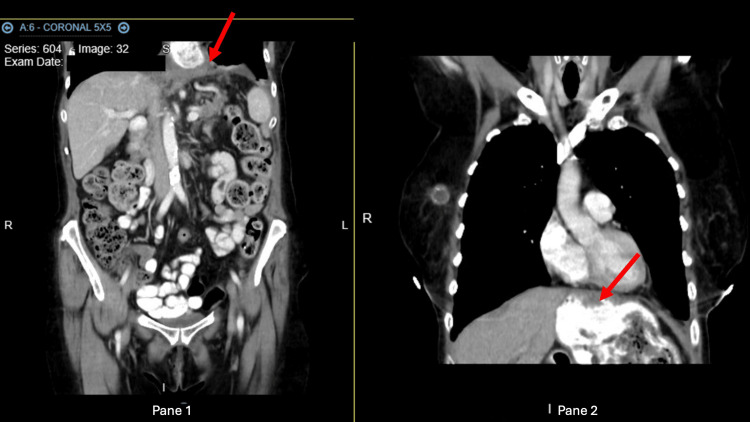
CT scan taken 113 days after open paraesophageal repair Findings: moderate hiatal hernia and moderate left pleural effusion CT: computed tomography
